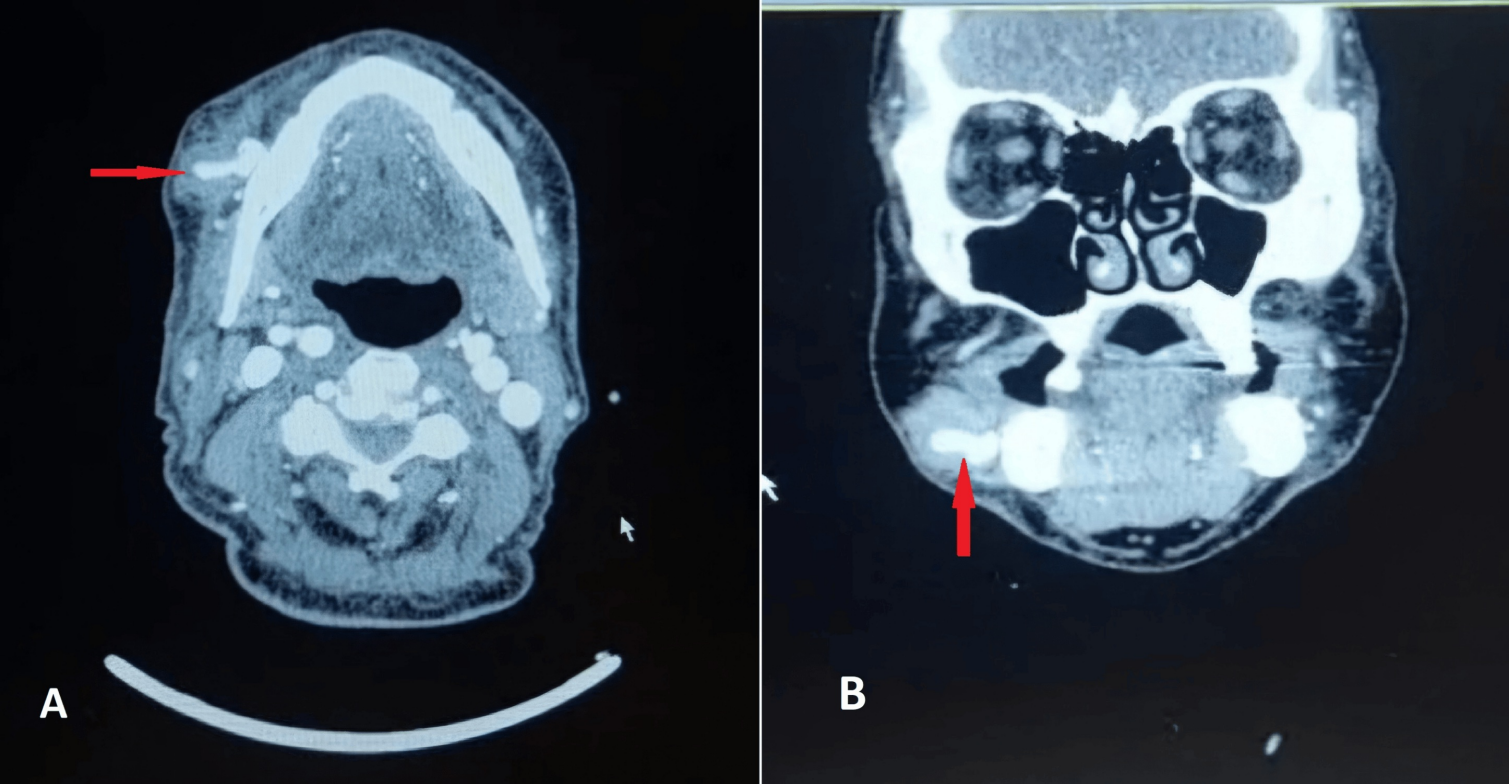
Pseudoaneurysm of the facial artery depicted in CT angiography, shown in axial (A) and coronal (B) planes
